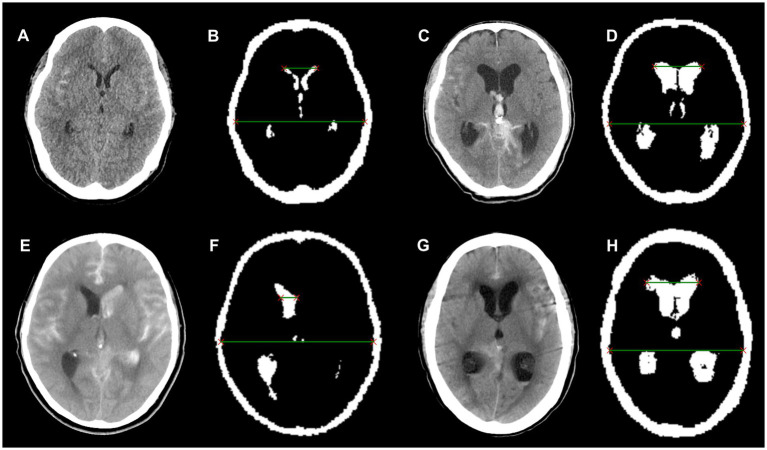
Representative examples of automated Evans index (EI) measurement under different ventricular and hemorrhagic conditions. Axial non-contrast head CT images (A,C,E,G) and the corresponding automated segmentations with Evans index measurement overlays generated by TotalSegmentator (B,D,F,H) are shown. (A,B) A case without ventricular enlargement. (C,D) A case with ventricular enlargement without frontal horn hemorrhage. (E,F) A case with left frontal horn casting by intraventricular hemorrhage. (G,H) A case with ventricular enlargement accompanied by periventricular exudation.
